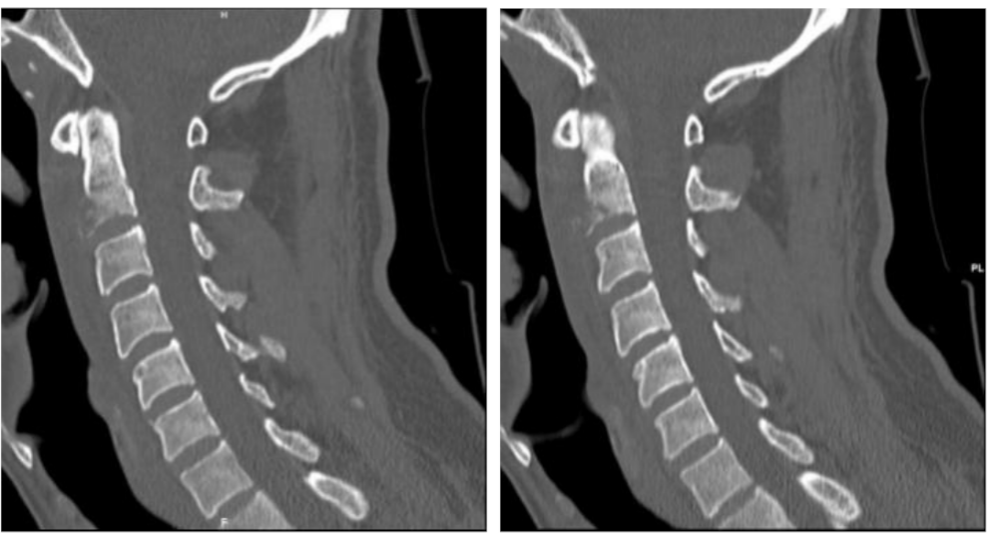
CT scan showing a destruction of the endplate and a loss of the trabecular structure in C2 vertebral body
